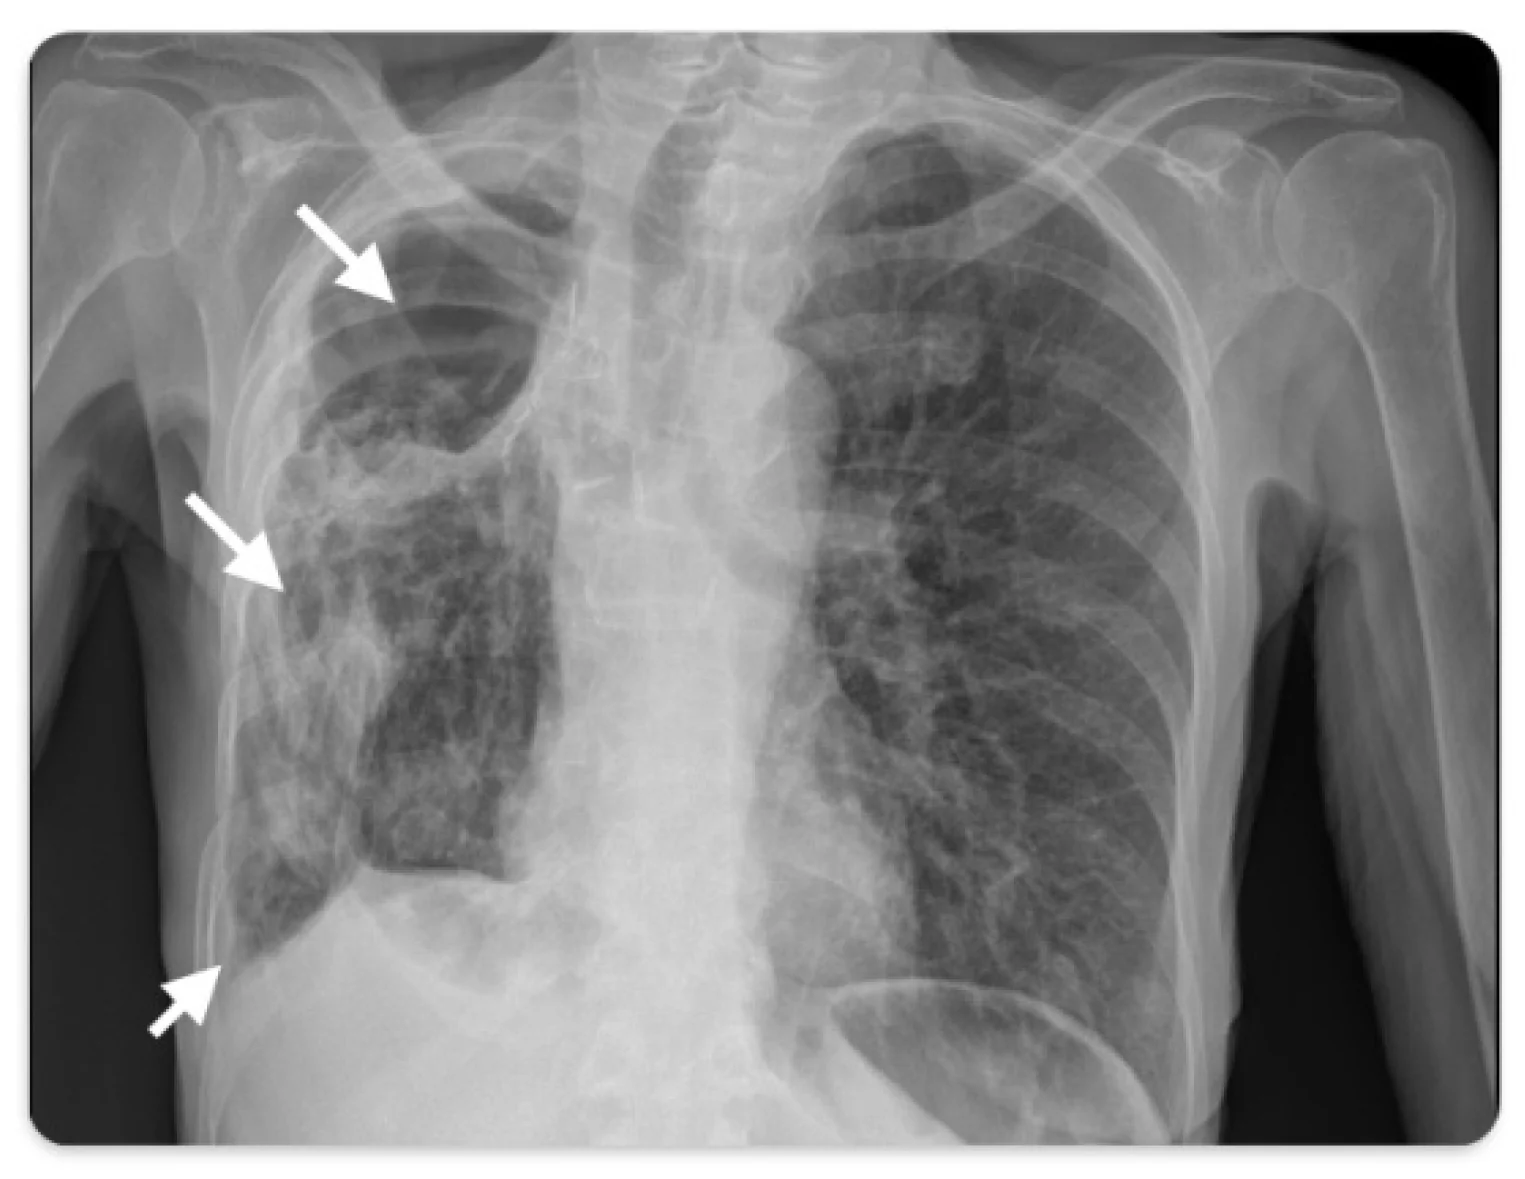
Chest X-ray showing hyperlucency of the right upper lung. Extensive consolidation involving the right middle and lower lung, as well as minimal right pleural effusion (see white arrows in the image).
